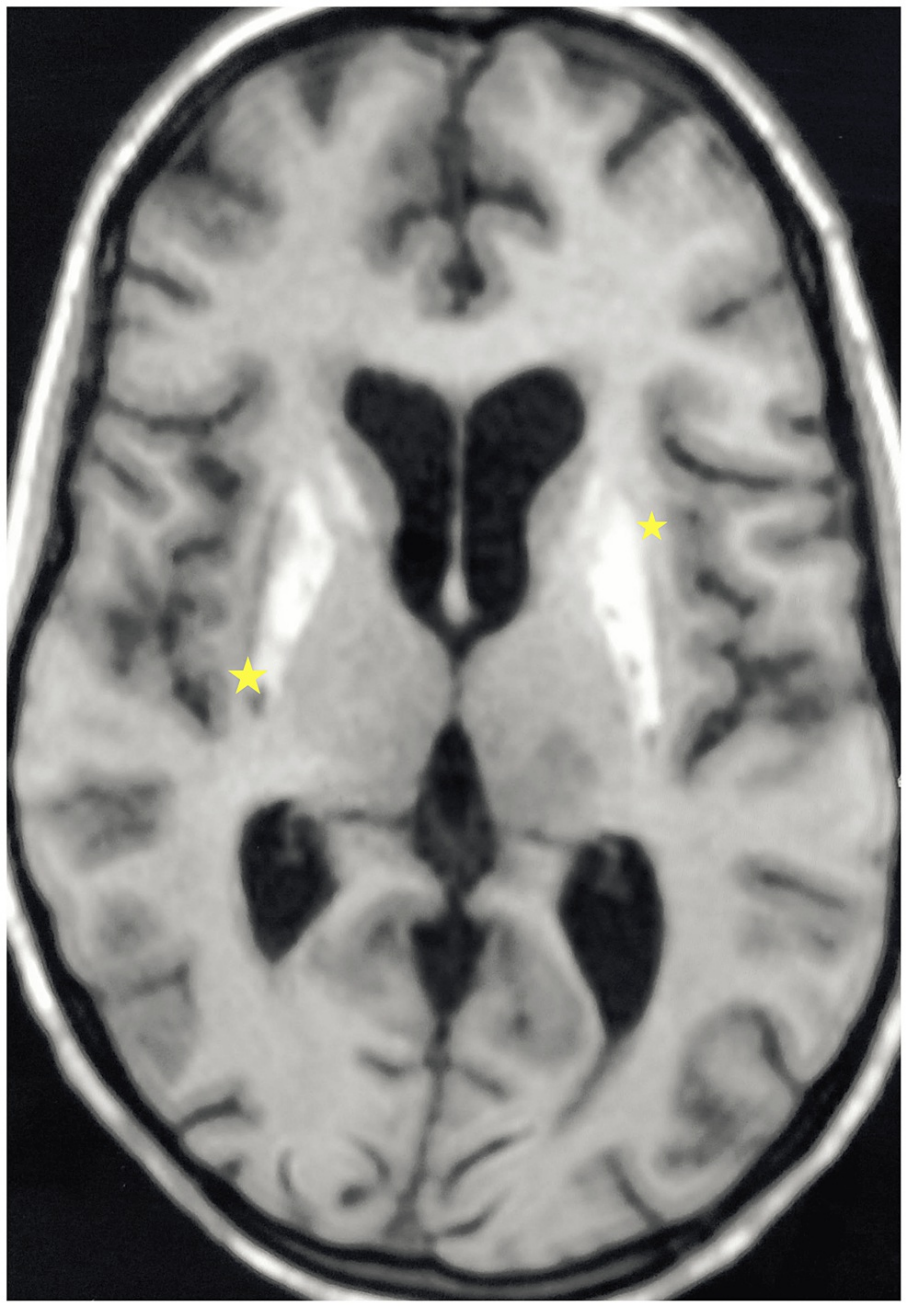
T1-weighted axial non-contrast MRI brain showing bilateral patchy hyperintense signals in bilateral basal ganglia (yellow star) regions suggestive of calcification
